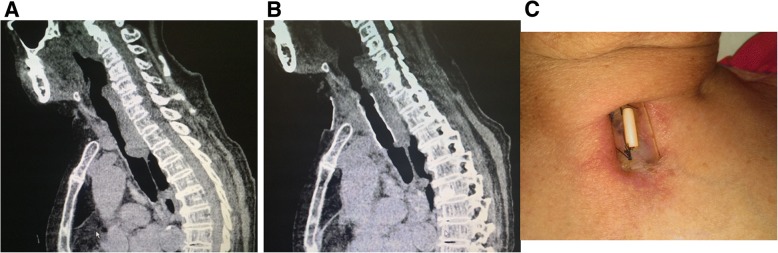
Preoperative and postoperative images of one patient (Case No. 1). a: Preoperative sagittal CT showing the upper tracheal stenosis. b: Postoperative sagittal CT showing the normal trachea. c: The appearance of the suture fixation on the neck
